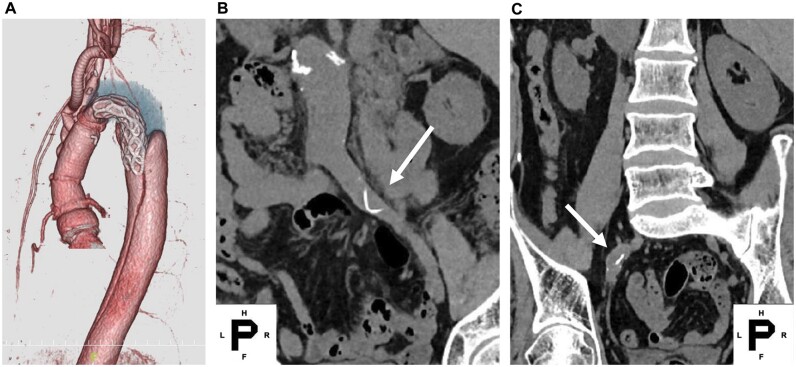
(A) Enhanced computed tomography scan showing the Bentall operation and total arch replacement with the flexion of the Frozenix J graft. (B) Computed tomography scan showing intravascular shadows of metal pieces in the right common iliac artery (arrow). (C) Computed tomography scan showing intravascular shadows of metal pieces in the left internal iliac artery (arrow).
